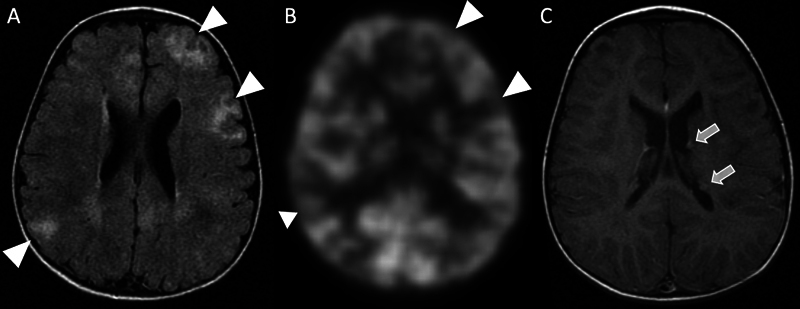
Brain MRI in a patient with tuberous sclerosis demonstrates multiple FLAIR hyperintense cortical tubers (
